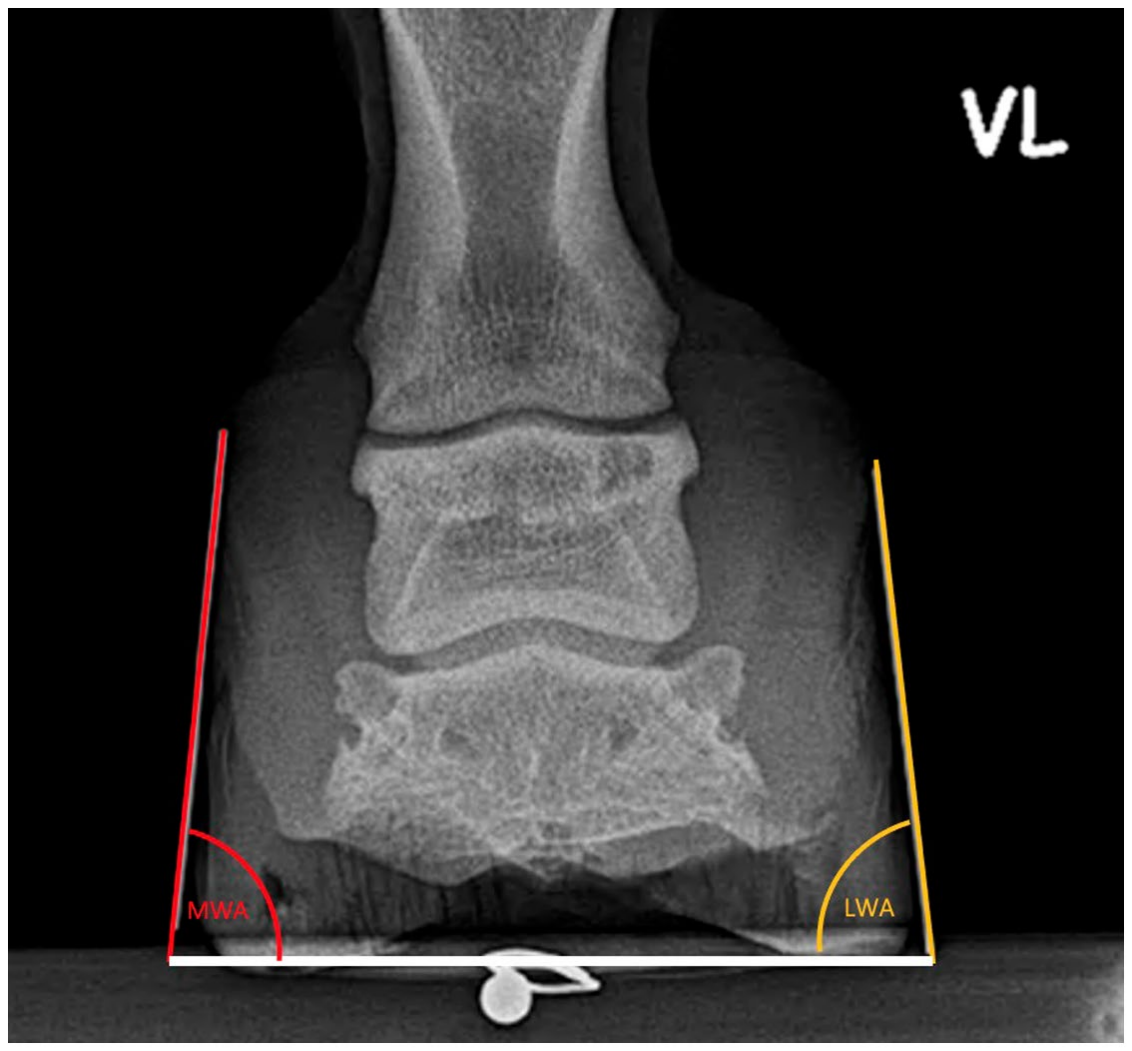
Angular measurements on the dorsopalmar X-Ray.
